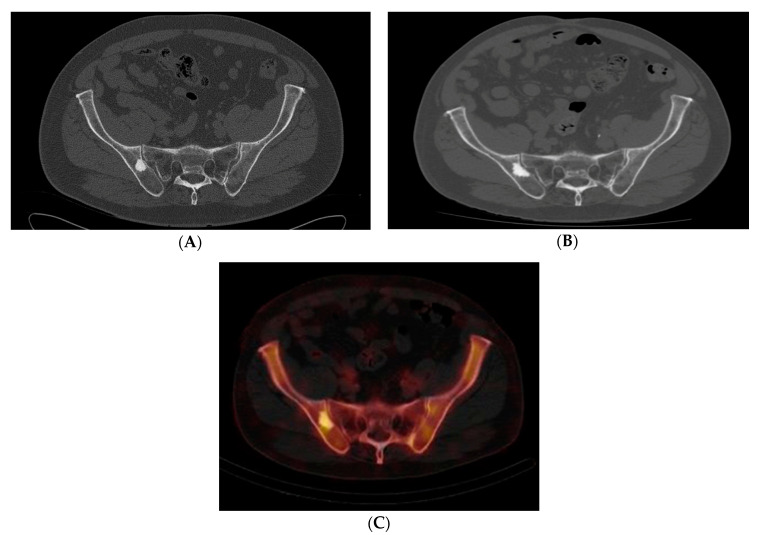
(A–C) Case 3. Pelvic axial CT shows an enlarged lesion 10 years after original discovery, which also demonstrated mild radiotracer uptake. (A) Initial pelvic axial CT shows an 18 mm sclerotic lesion in the right ilium near the SI joint. (B) Repeat pelvic CT 10 years later shows enlargement of the lesion to 27 mm. (C) Axial SPECT-CT Tc-99m bone scan demonstrated mildly increased radiotracer in the right iliac bone correlating with the region of the patient’s enlarging bone lesion. Although low-grade central osteosarcoma or osteoblastoma could not be ruled out, we believed atypical BI was the most likely diagnosis. The patient opted for observation rather than bone biopsy. There has been no routine follow up visits over the last 10 months.
